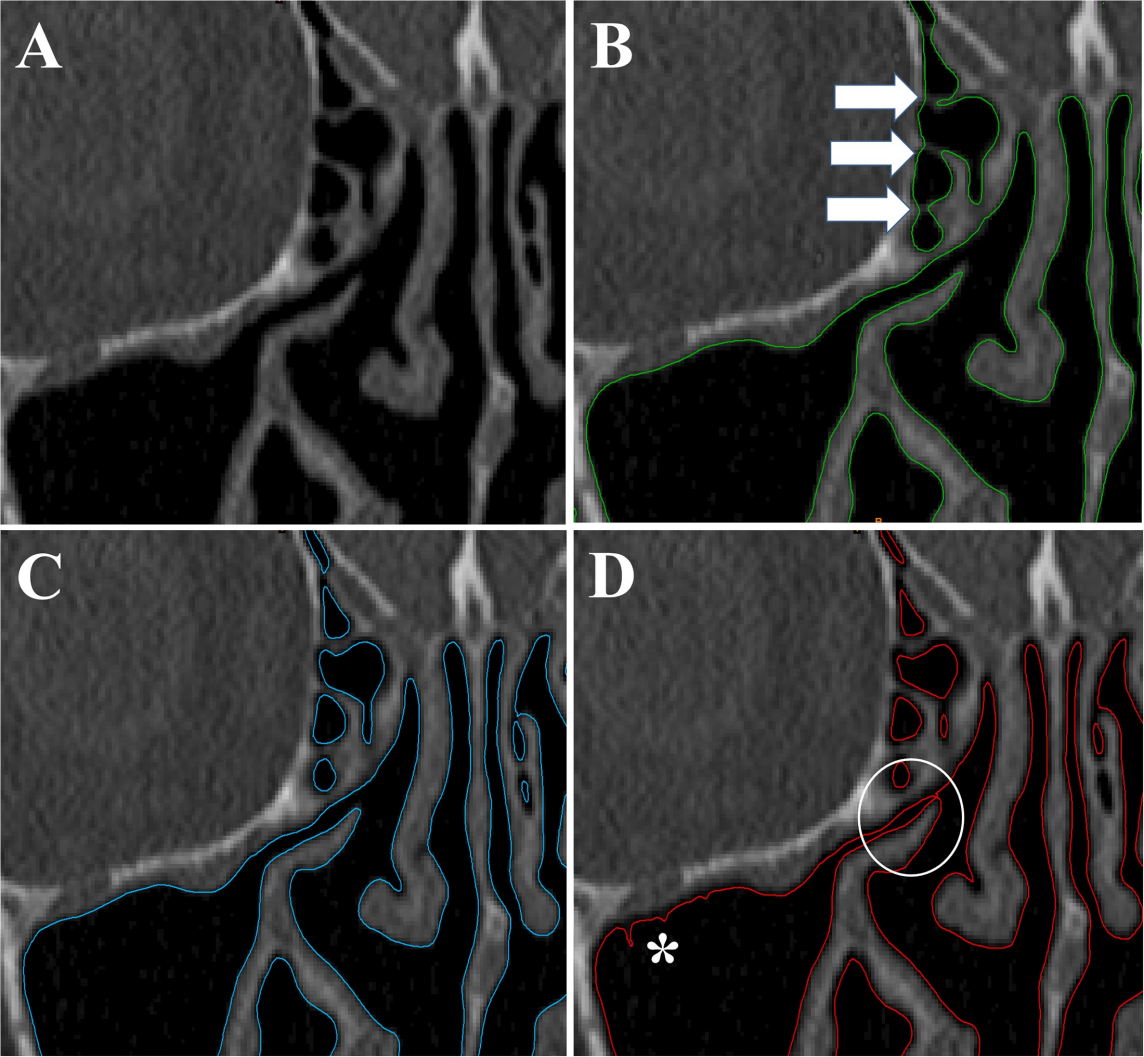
Effects of segmentation threshold on 3D reconstruction of the nasal cavity. A wide range of segmentation thresholds (namely -800HU to -300HU) provides acceptable 3D reconstructions of the human nasal cavity. (A) Coronal CT of patient #1 after mucosal decongestion showing the middle turbinate, ethmoid sinuses, and part of the right maxillary sinus. (B,C,D) Contours of 3D models created using three different thresholds (-300 HU, -550 HU and, -800 HU). At the upper limit of acceptable thresholds (-300 HU, panel B), thin soft tissue walls are incorrectly identified as air (arrows). At the center of the range (-550 HU, panel C), a good 3D reconstruction is obtained with few or no artifacts. At the lower limit of acceptable thresholds (-800HU, panel D), narrow passages become partially or completely obstructed (circle) and irregularities appears at air-tissue boundary (asterisk).
